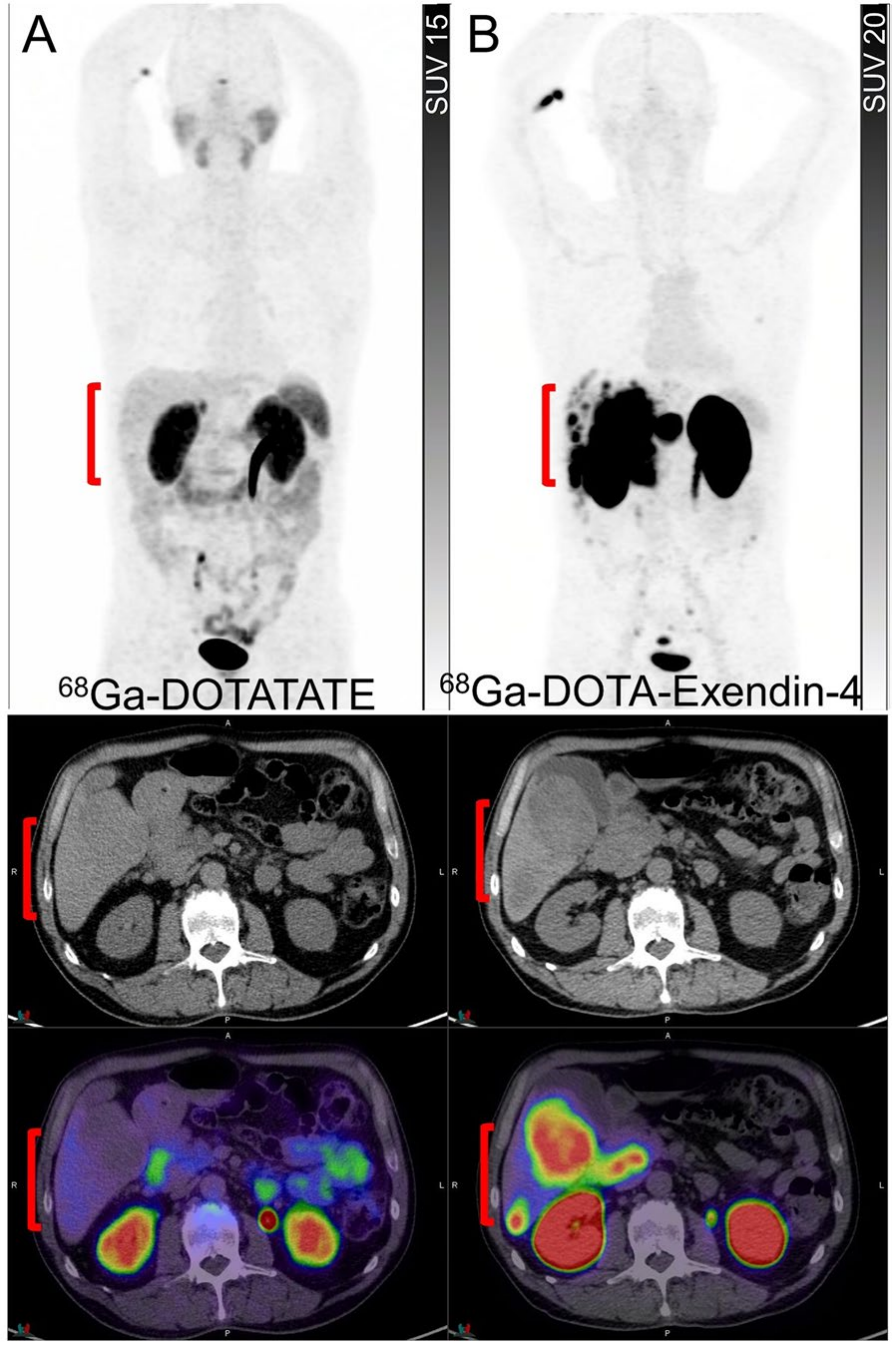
A patient with well-differentiated duodenal NET (G1, Ki67 < 2%) underwent ^68^Ga-DOTATATE for the assessment of suitability for ^177^Lu-DOTATATE. ^68^Ga-DOTATATE PET CT and PET/CT (A) showed no uptake in the hepatic metastases (brackets). ^68^Ga-DOTA-Exendin-4 PET, CT and PET/CT (B) showed intense uptake in the hepatic metastases (brackets)
